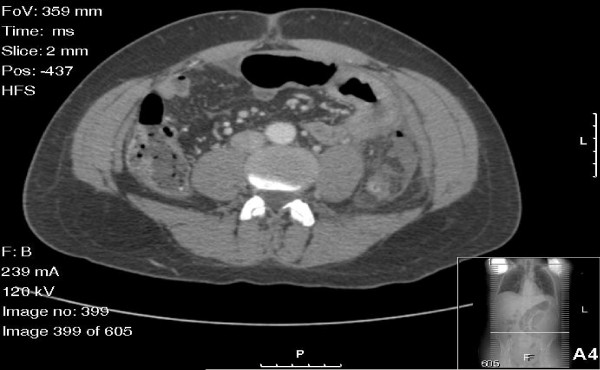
Computed tomographic scan of the patient's abdomen showing pockets of free air and fluid in proximity to the jejunum and a thickened left colon with inflammatory stranding. Inflammatory changes are present in the peritoneal fat, especially adjacent to the left colon. These findings are consistent with a perforated sigmoid colon.
